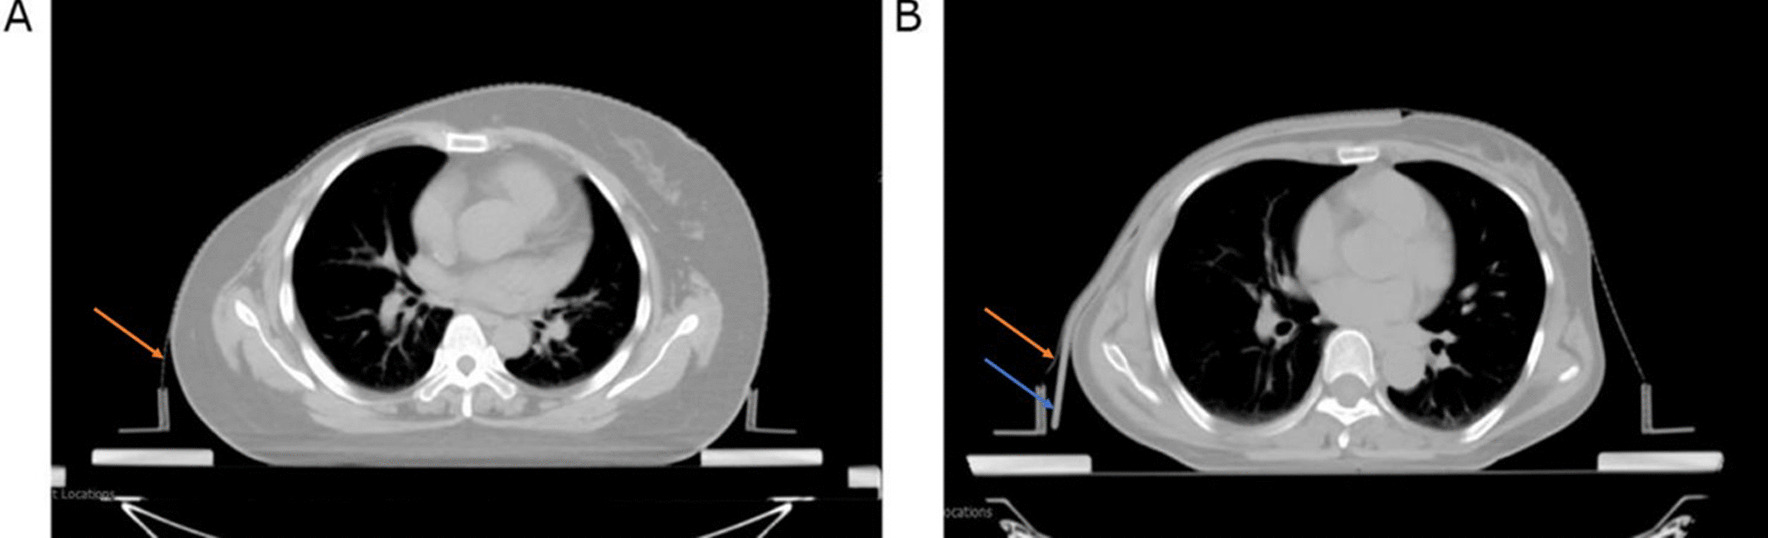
CT scan images with and without bolus application. A Thermoplastic shell was used to immobilize the patient's position during the scan; B Bolus was applied between the thermoplastic shell and chest wall.
